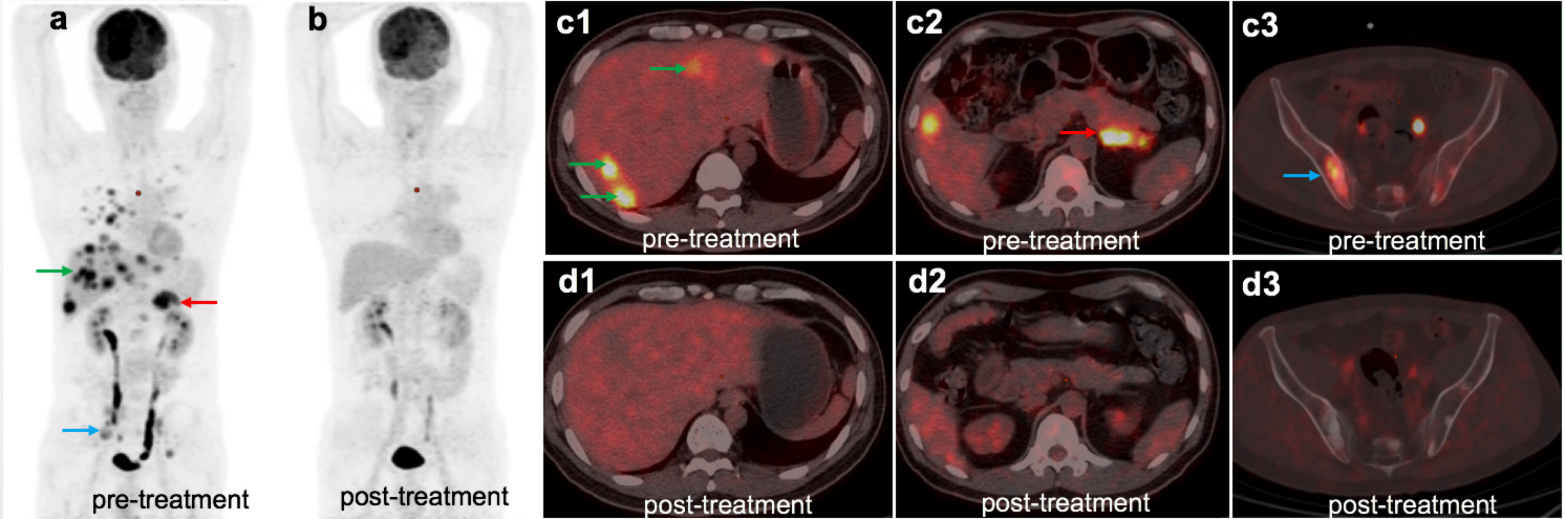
The patient underwent 18F-FDG PET/CT scan before (a) and after (b) anti-PD-1 treatment, the figure above shows the whole body maximum intensity projection PET and representative PET/CT fused axial images. 18F-FDG PET/CT scan revealed NPC with metastases in multiple organs, including the liver (c1), right adrenal (c2) and pelvis (c3). After receiving 5 cycles of pembrolizumab, all metastatic lesions mostly disappeared (d1, d2, d3).
